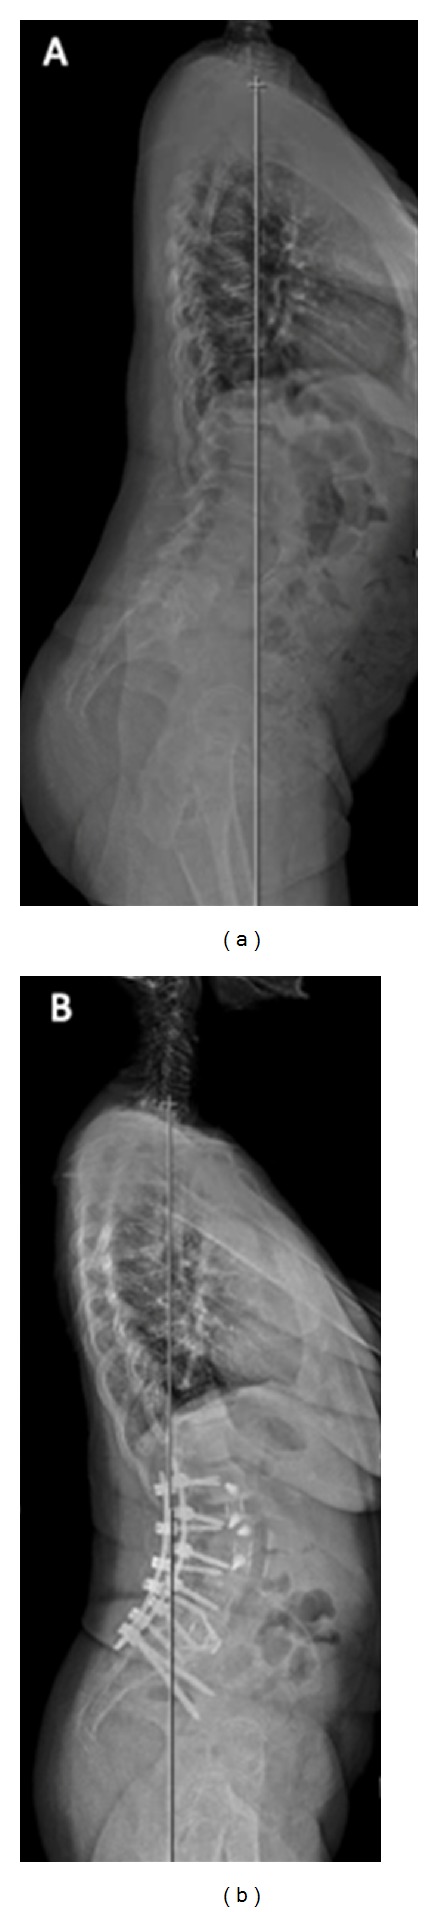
(a) Pre- and (b) postoperative standing 36 inch lateral radiographs demonstrating improvement in sagittal vertical axis after two-level ALR plus multilevel MIS LIF with open posterior instrumentation.
